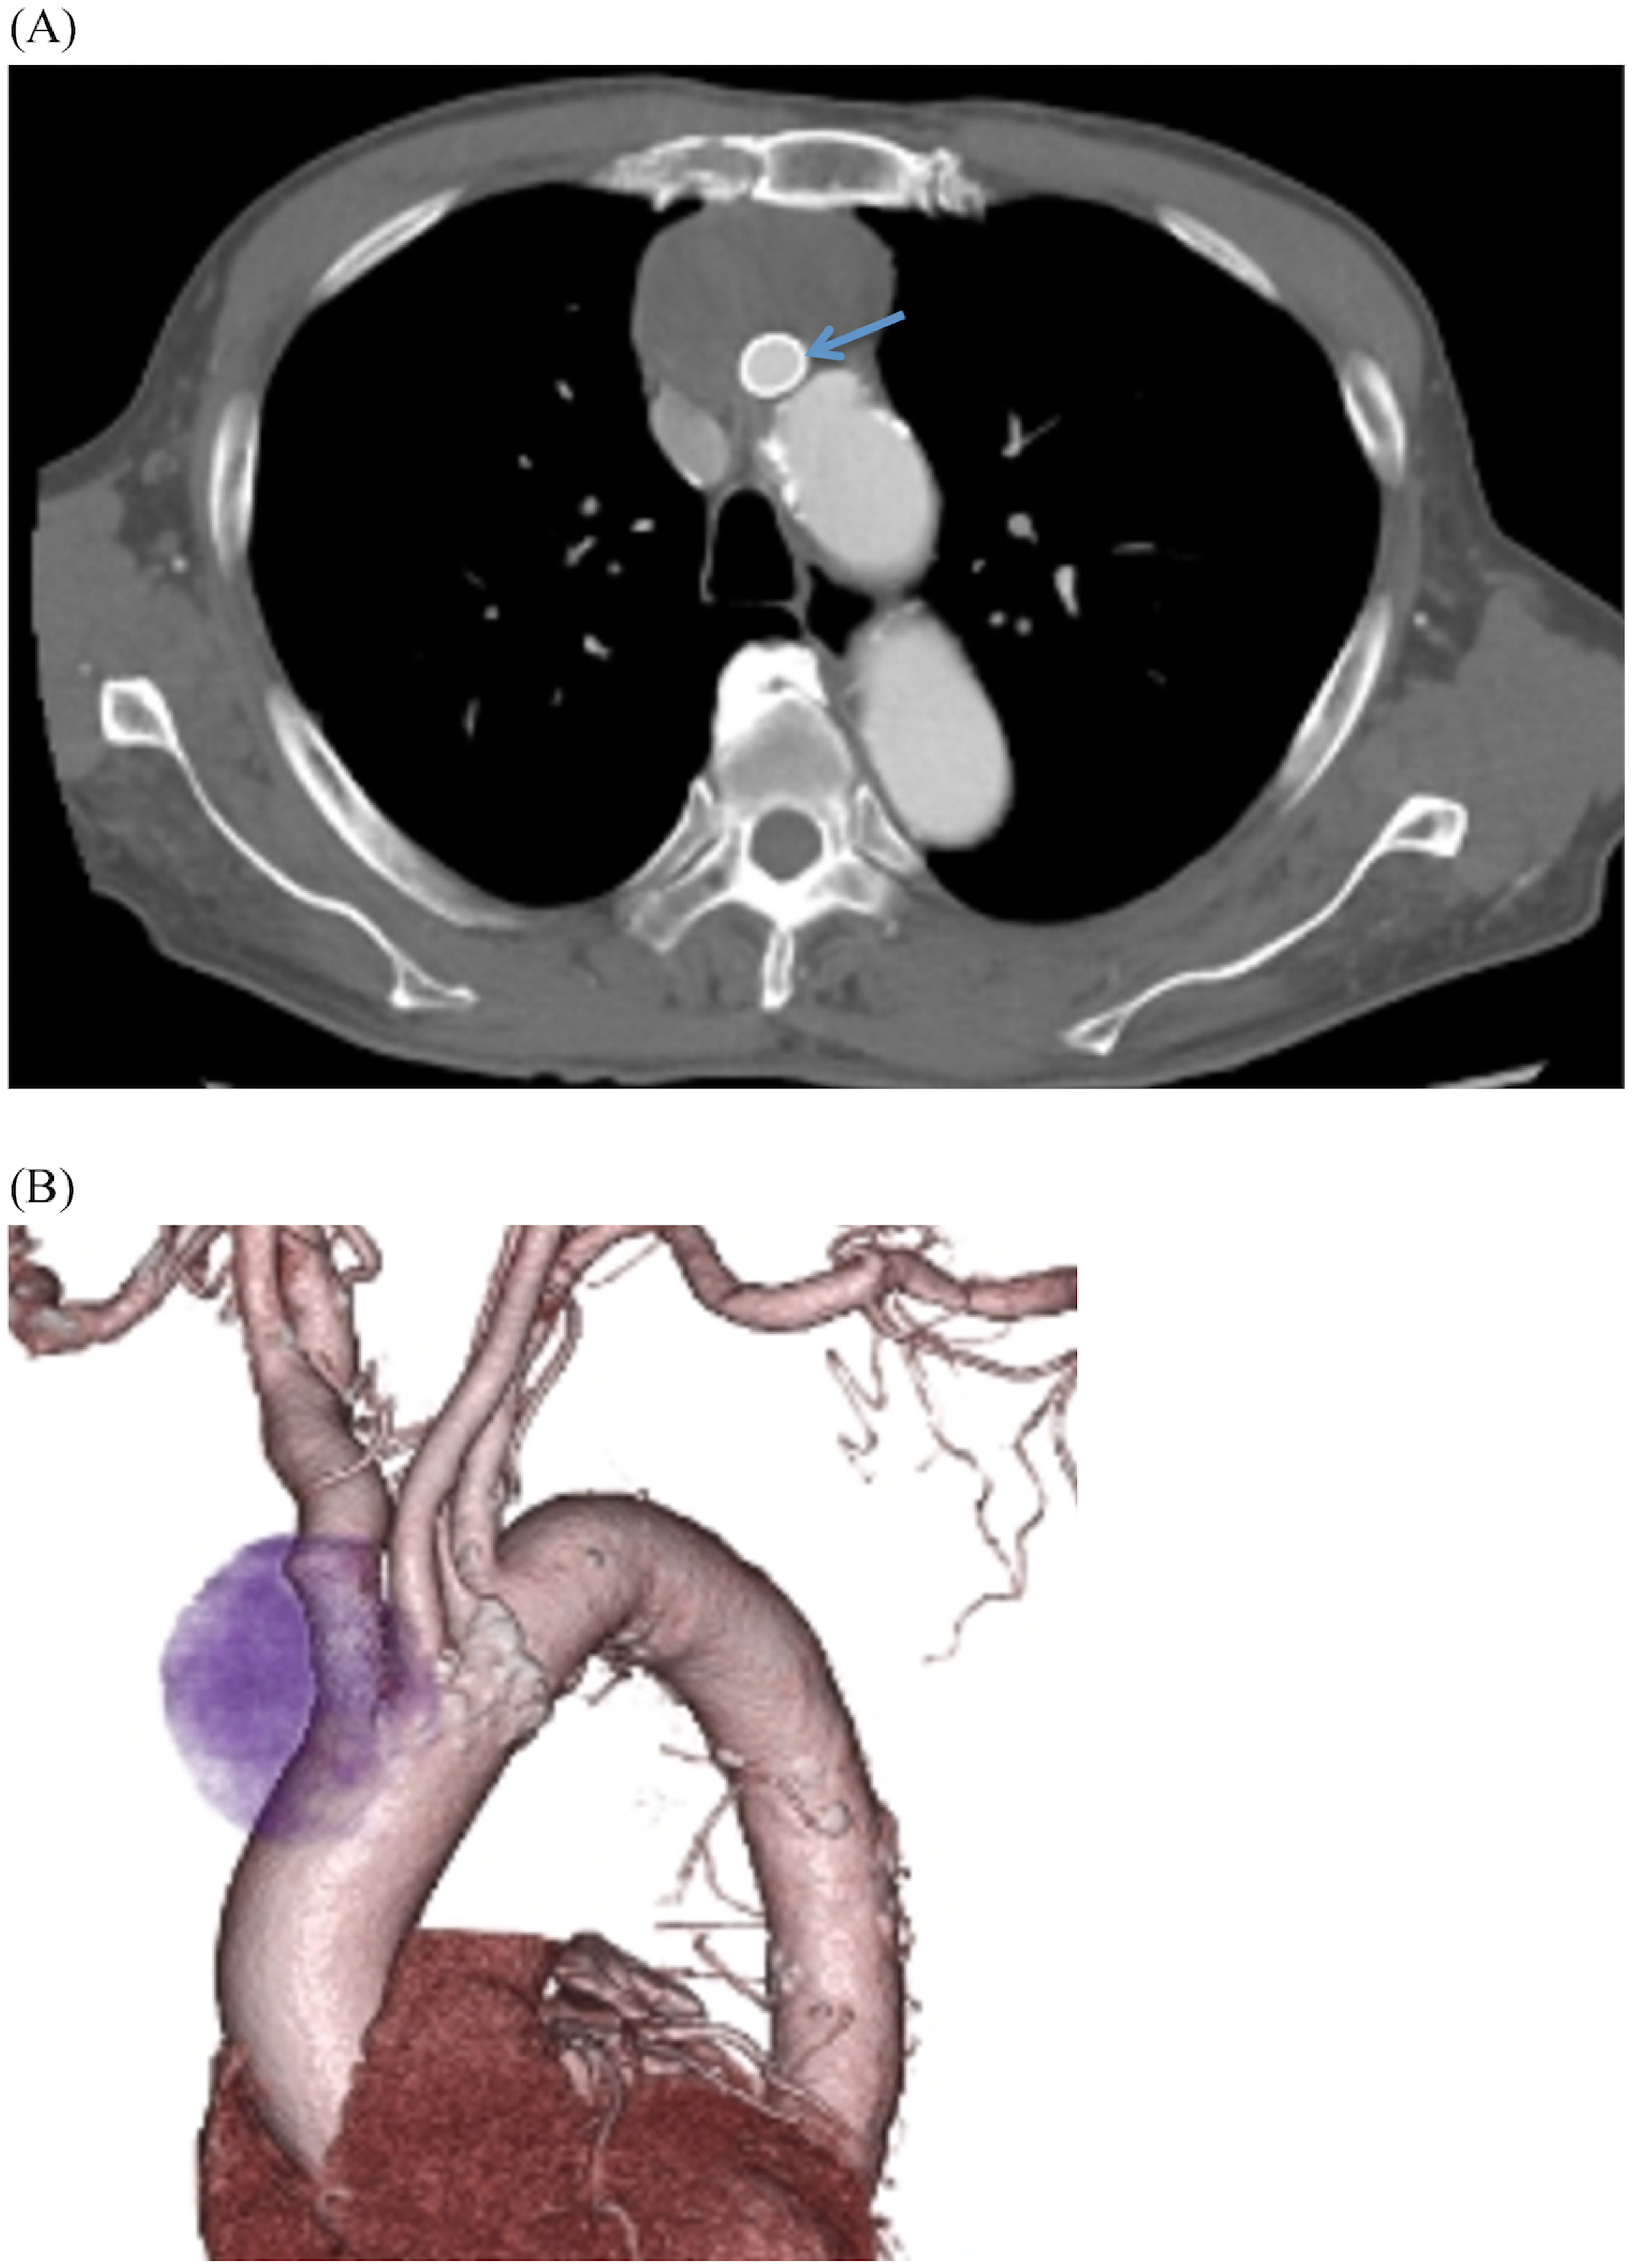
Fig. 3 Postoperative computed tomography images. (A) Coronal view showing the disappearance of contrast leakage and uncompressing of the brachiocephalic artery (arrow). (B) A three-dimensional constructed image shows the proper position of the VBX.
